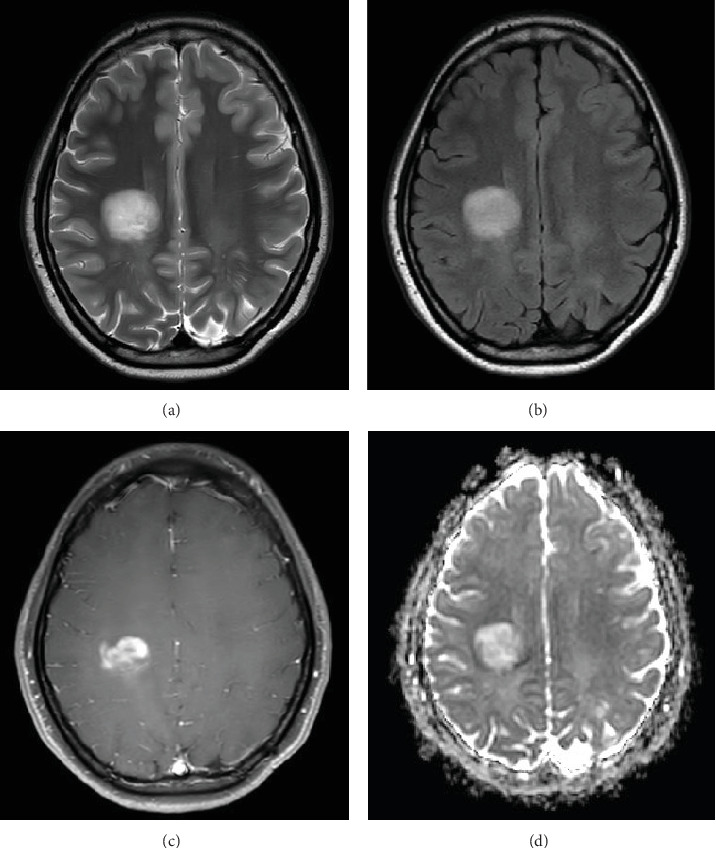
Axial images from brain MRI (on the day of admission) demonstrating a focal, mildly heterogeneous, 2.9 × 2.4 cm hyperintense area of signal abnormality on (a) T2 TSE and (b) T2 FLAIR. On the postcontrast T1-weighted image (c), there is a patchy, heterogeneous enhancement within the lesion. DWI ADC map (d) reveals areas of low ADC values within and around the periphery of this lesion.
